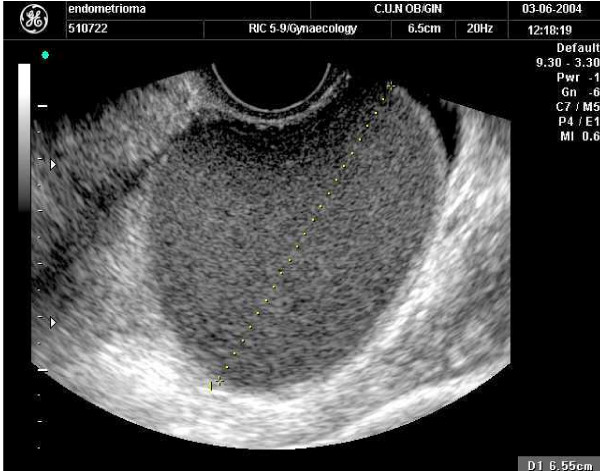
Ultrasound imaging of the ovarian cystic lesion.
